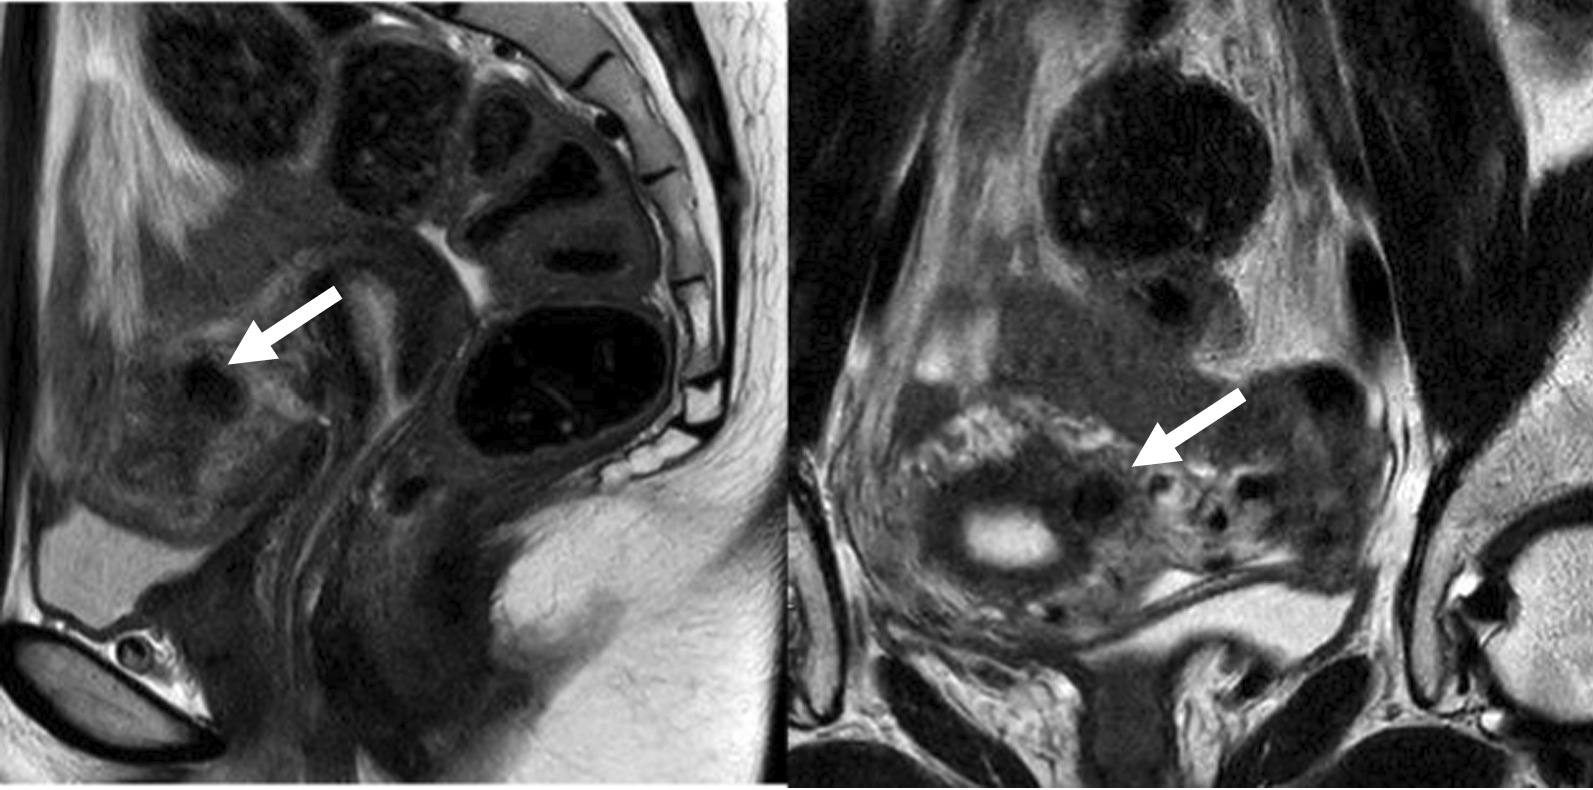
MRI showing an intramural fibroid in contact with the endometrium
